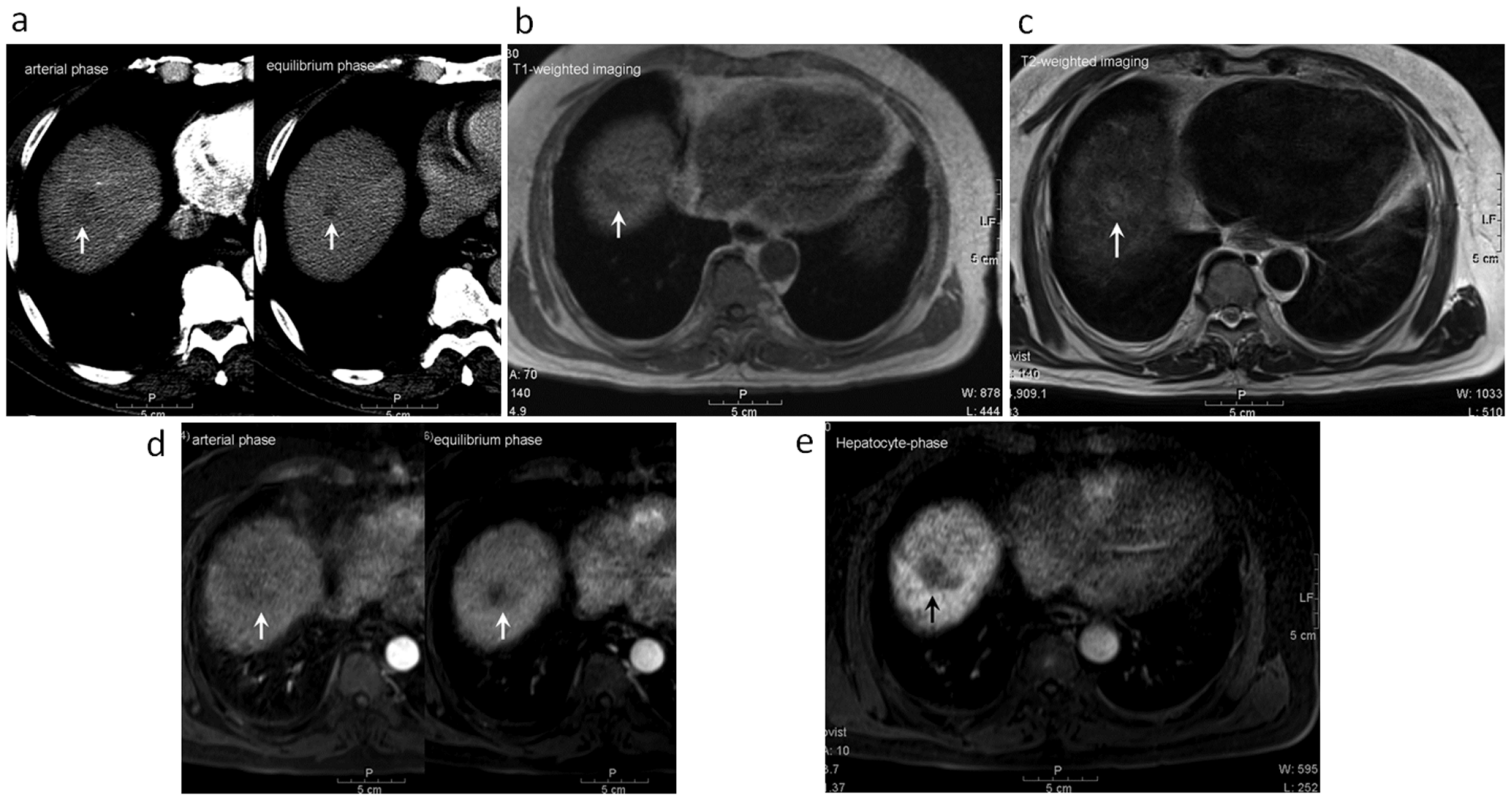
A 57-year-old male with a well-differentiated hepatocellular carcinoma (indicated by arrow) at S8 of the liver underwent gadoxetic acid-enhanced MR imaging and liver biopsy. (a) The tumor depicted hypovascular enhancement in the arterial-phase image and isodensity to hypodensity relative to the adjacent liver parenchyma on equilibrium-phase dynamic CT study. (b) The tumor showed hypointensity on the T1-weighted image. (c) The tumor showed isointensity to hyperintensity on T2-weighted image. (d) The tumor also showed hypovascular enhancement in arterial-phase imaging and hypointensity relative to the adjacent liver parenchyma on equilibrium phases of dynamic MR study. (e) The tumor showed hypointensity on the gadoxetic acid-enhanced hepatocyte-phase T1-weighted image.
